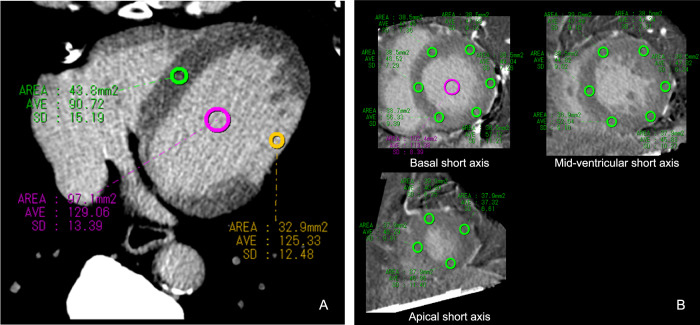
Quantitative evaluation of image quality involves placing circular ROIs in the MI segment (yellow), the non-MI segment (green), and the LVC (pink) on the axial plane to measure the SNR and the CNR (A). To evaluate global and segmental ECV, a circular ROI is positioned in each myocardial segment and LVC across three short-axis planes: basal, mid-ventricular, and apical subtraction images (shuttle mode) or iodine-specific images (dual-energy mode) (B). For clarity, A and B utilize different cases, both captured in shuttle mode. A features a myocardial CT late enhancement image, whereas B presents subtraction images. ROI, region of interest; MI, myocardial infarction; SNR, signal-to-noise ratio; CNR, contrast-to-noise ratio; LVC, left ventricular cavity; ECV, extracellular volume
